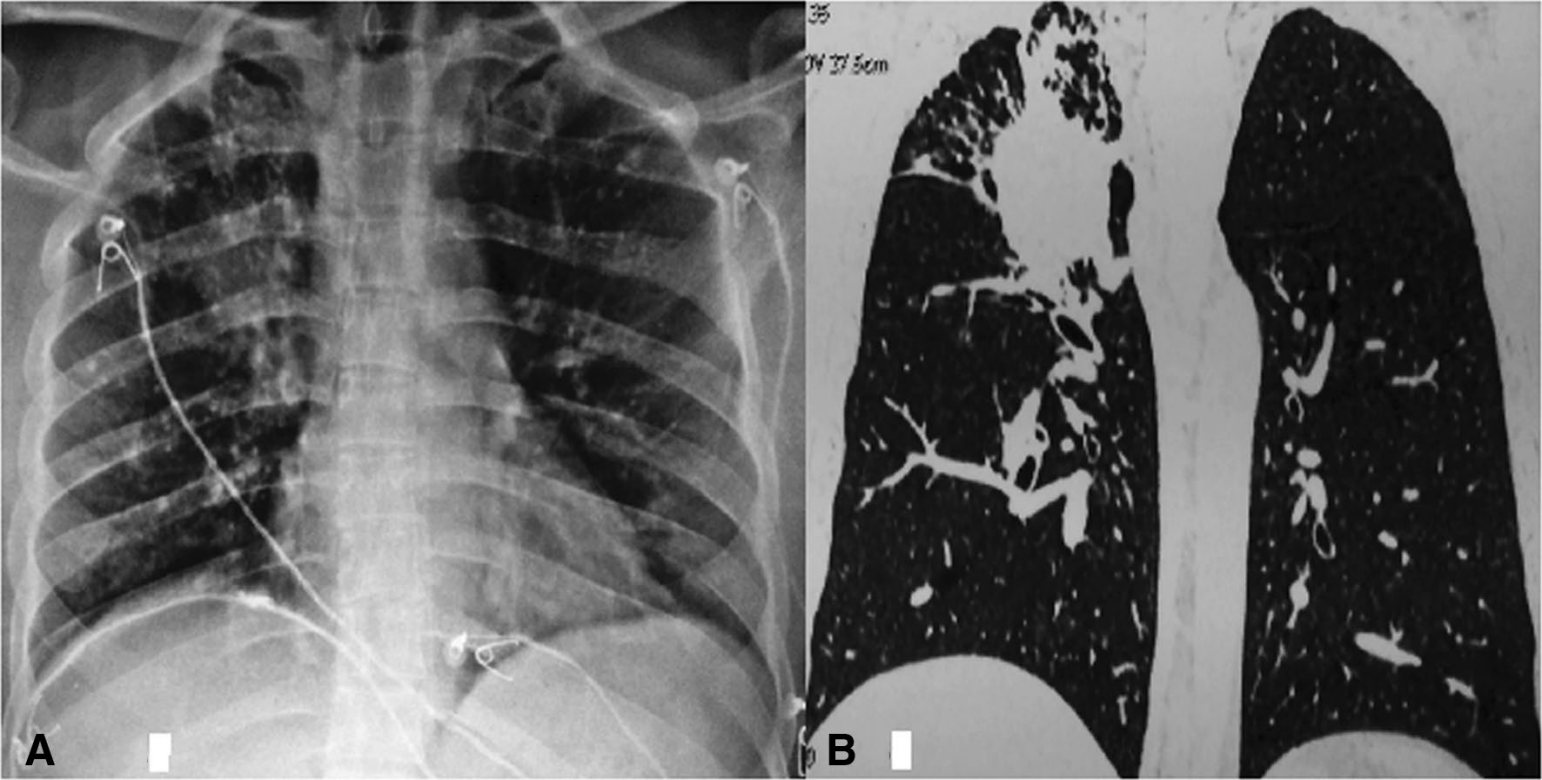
A Chest Xray showing opacity *right upper* lobe B HRCT sagittal cut showing expanded aspergilloma pushing across oblique fissure with peripheral infiltrates
